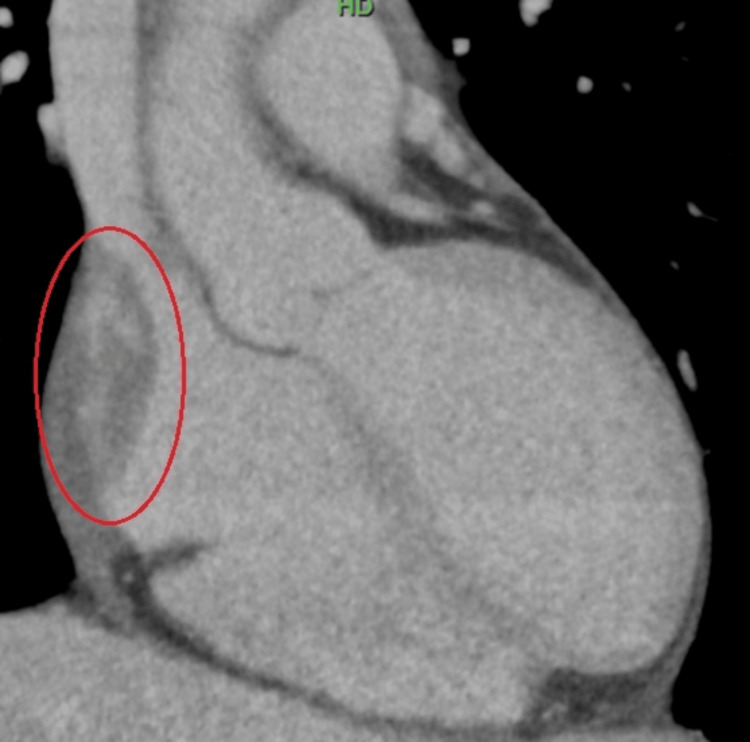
Preoperative cardiac computed tomography (CT). CT chest showing an irregular filling defect (red oval) in the infero-posterior aspect of the right atrium adjacent to the inferior vena cava, extending superiorly to the junction of the superior vena cava, measuring up to 4.5 cm in length (from the superior vena cava (SVC) junction to the inferior right atrium) and 1.5 cm in diameter.
